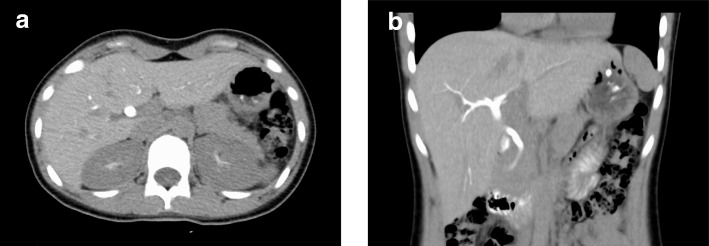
Drip infusion cholecystocholangiography with computed tomography (DIC–CT) performed at 6 weeks after discharge. The bile ducts were analyzed by DIC-CT using a radiopaque dye as a contrast medium. We intravenously administered the dye, which accumulates preferentially in the tissue of gallbladder or bile ducts. The dye did not show any silhouette of the gallbladder, indicating the absence of the gallbladder. a Axial view, b coronal view
